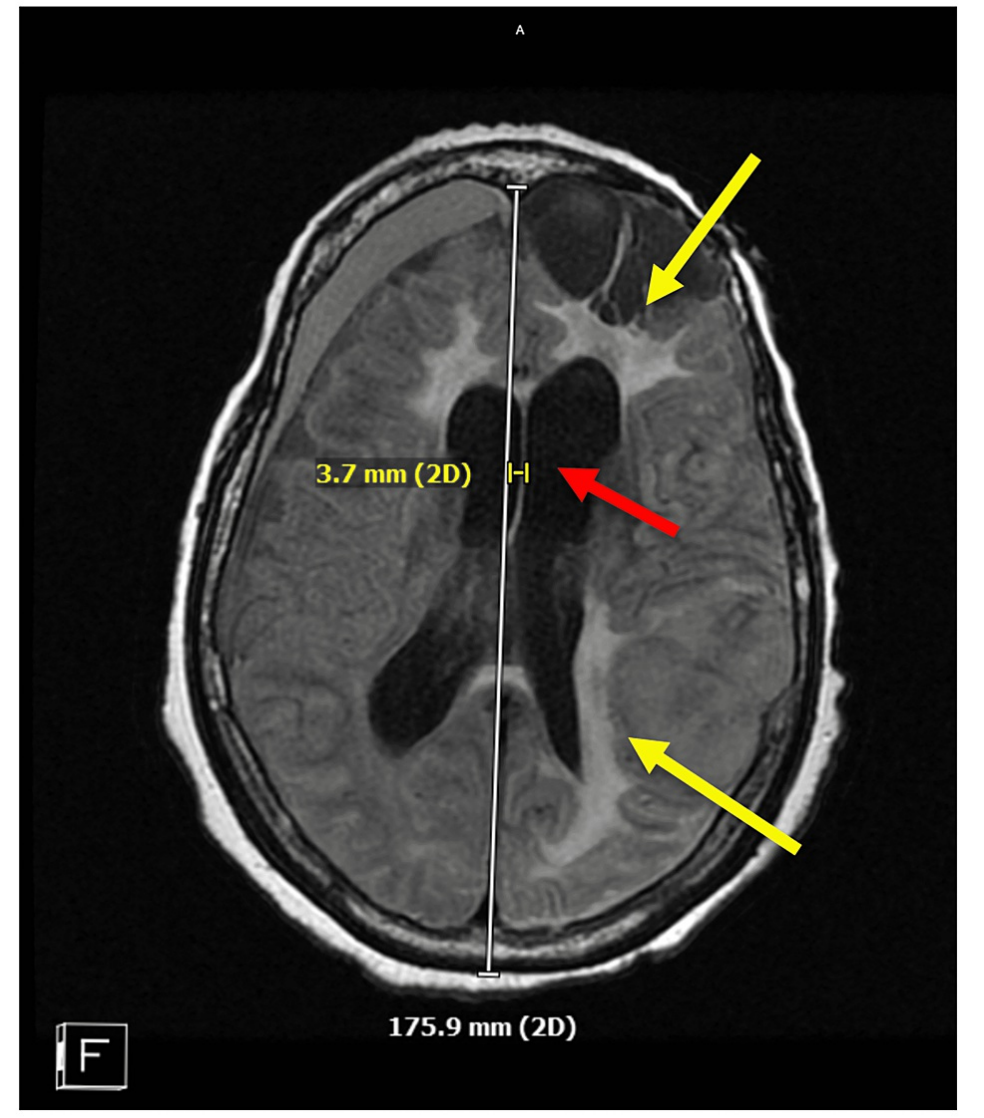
MRI of the patient's brain in transverse view demonstrating chronic changes In this transverse view, a 3.7-mm midline shift is seen (red arrow), likely from the subdural hematoma. There are also areas of edema noted (yellow arrows).
